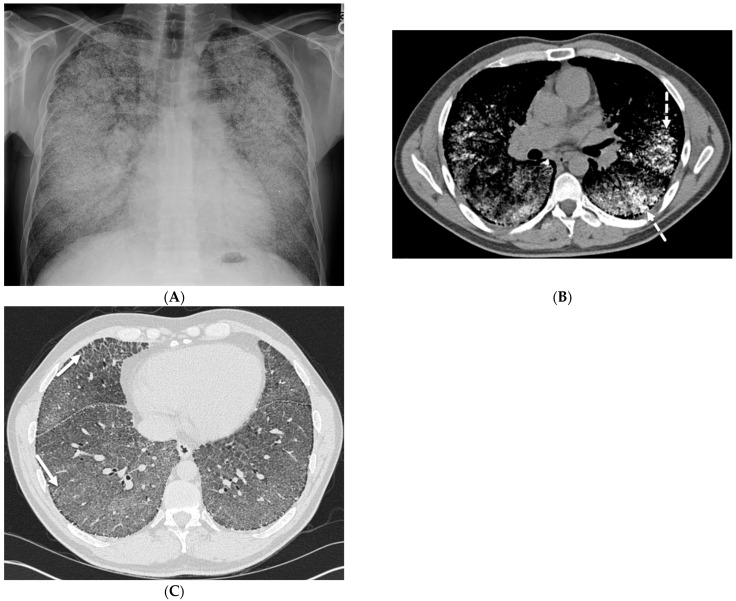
A 42-year-old male with a history of pulmonary alveolar microlithiasis. A frontal chest radiograph (A) and axial chest CT images (B,C) show a diffuse increase in pulmonary density with more confluent areas of calcification/ossification in both upper and mid lung zones (dashed white arrow). Interlobular septal thickening is noted in both lung bases (solid white arrow).
